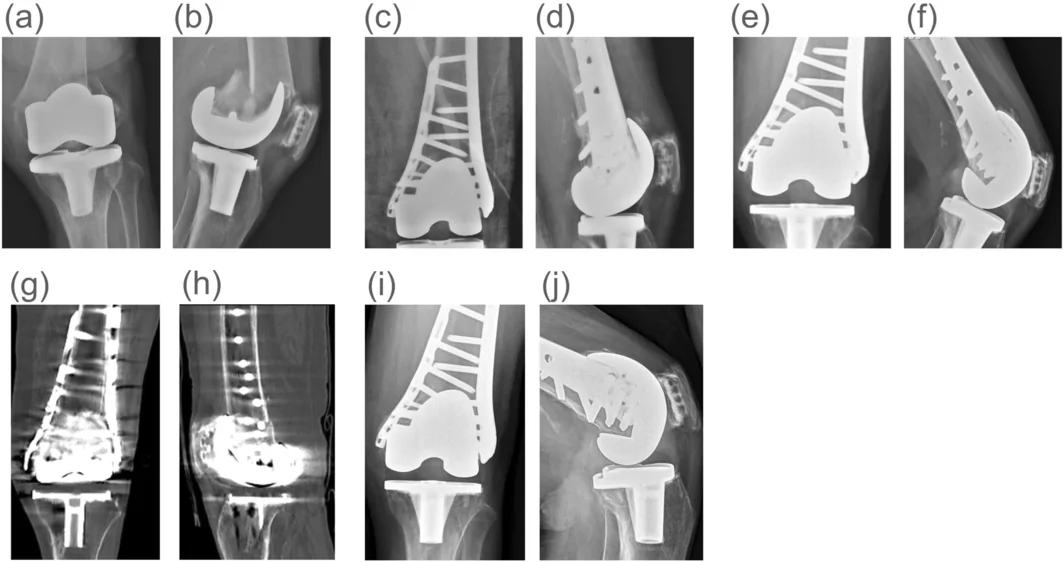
An 89‐year‐old woman with a left distal femoral periprosthetic fracture after a fall. (a) and (b) Preoperative radiographs obtained in the emergency department. (c) and (d) Postoperative radiographs after dual‐plate fixation. (e) and (f) Radiographic bony union at 3 months postoperatively. (g) and (h) CT confirmation of bony union at 6 months postoperatively. (i) and (j) Latest radiographic image at 18 months postoperatively.
